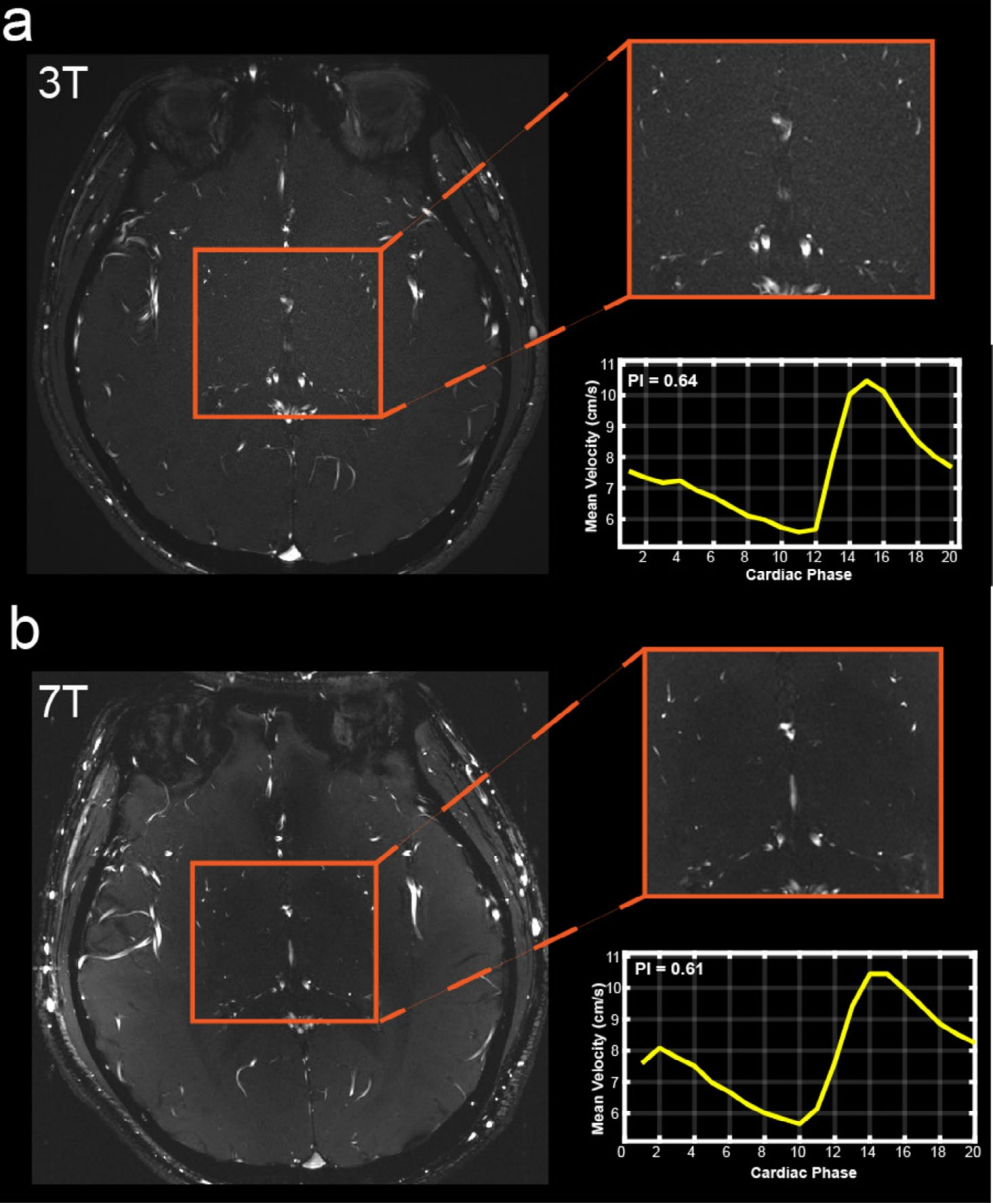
Low VENC (20 cm/s) magnitude images from a dual‐VENC PC‐MRI acquisition with zoomed‐in view showing detected perforators as well as the extracted velocity waveform at both 3T (a) and 7T (b) from the same representative case. The image contrast was adjusted using the window center and window width acquired from the DICOM information for better and direct visual assessment.
